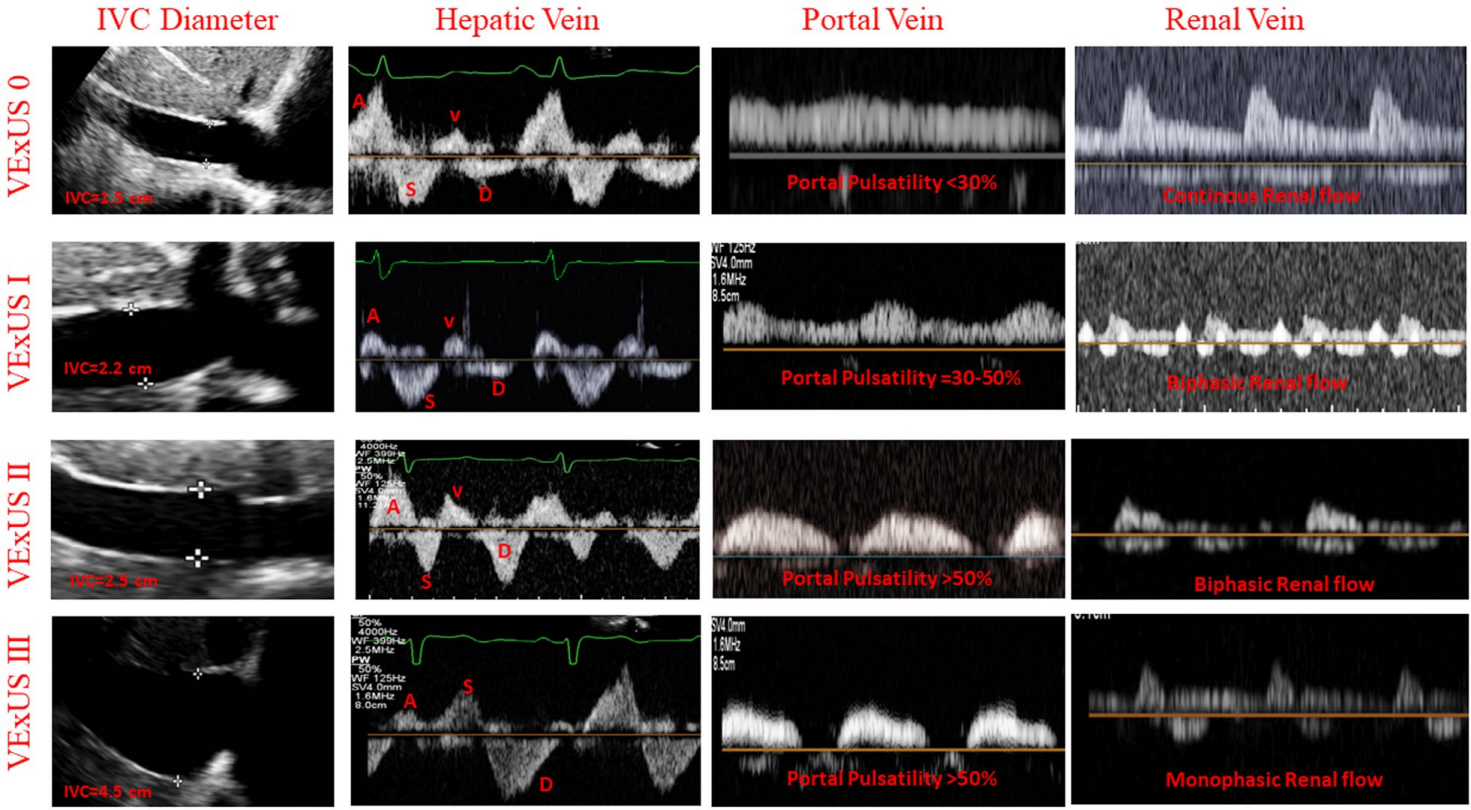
Clinical examples of venous congestion by VExUS score. Representative images demonstrating the ultrasound findings corresponding to different VExUS score grades
